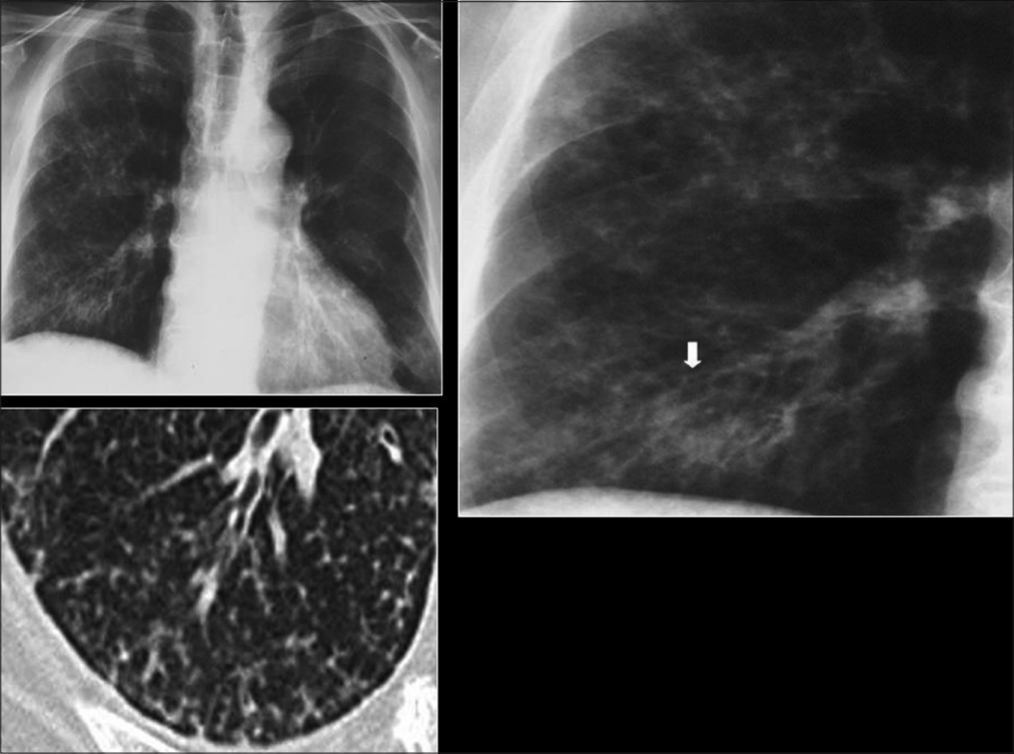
Bacterial bronchiolitis. Chest X-ray of a 36-year-old human immunodeficiency virus-positive male with a CD4 count of >400 presenting with productive cough. Note (left) the peribronchial thickening. The right hand film is a blown-up image showing the peribronchial thickening more clearly (arrow). The high-resolution computed tomography (lower left) depicts bronchiectasis, centrilobular nodularity/“tree-in-bud” mosaic attenuation in the same patient
